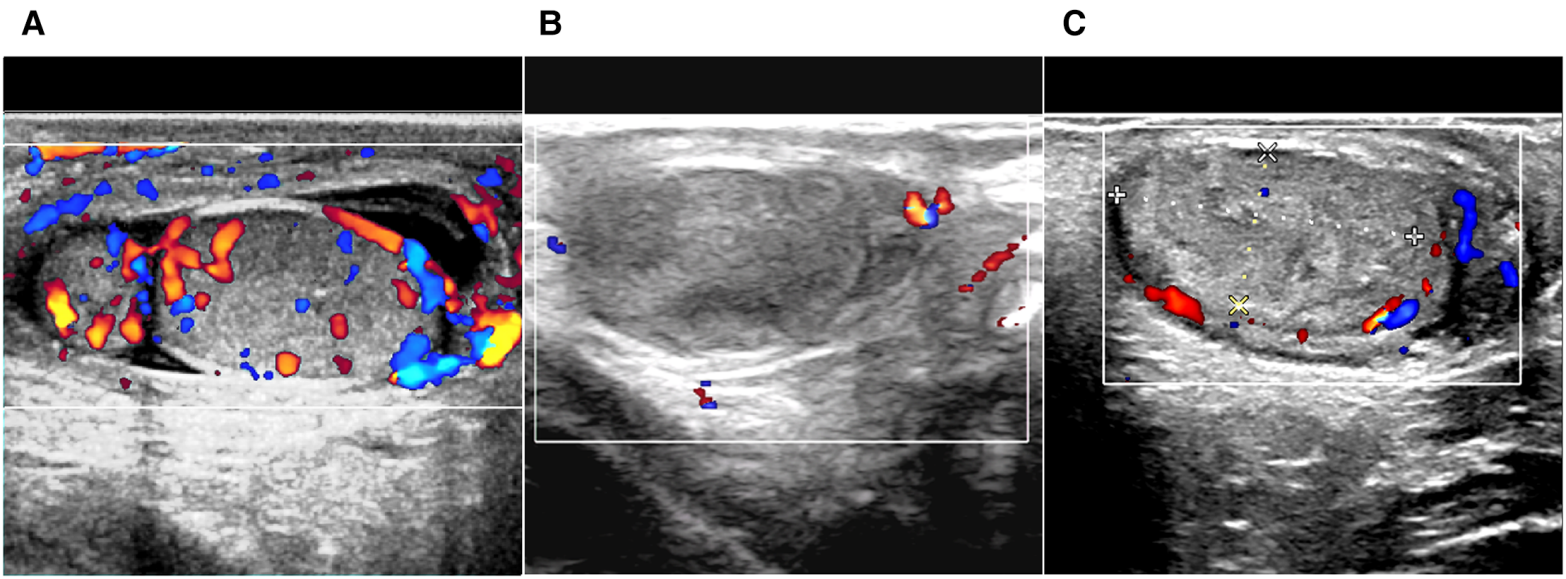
Ultrasound scan of immunoglobulin A vasculitis with testicular/epididymal involvement. (A) Enlarged epididymis, fluid retention in the scrotum, and increased blood flow in the testis are shown on color Doppler. (B,C) The testis has hyperechoic and heterogeneous echoic areas, and the intratesticular blood flow is absent (B) or decreased (C).
